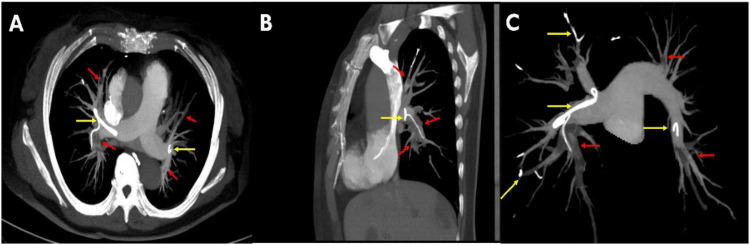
Computed tomography pulmonary angiography (CTPA) showed multiple low-density shadows in the primary and peripheral branches of both pulmonary arteries indicating thromboembolism (red arrows); A high density cord foreign body in the right atrium and pulmonary artery, multiple exuding foreign bodies in both side’s pulmonary branch arteries (yellow arrows). [(A) Axial view, (B) sagittal view, (C) coronal view].
